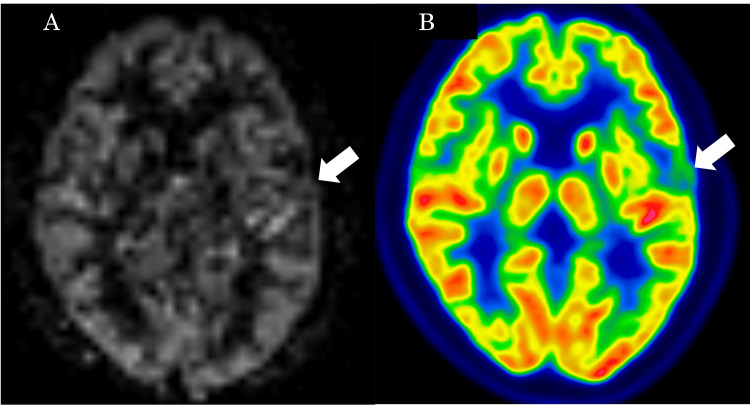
The ASL and FDG-PET scan Pseudo-continuous ASL (A) shows no obvious hyperperfusion within the tumor, and FDG-PET (B) shows no abnormal FDG uptake with a maximum standardized uptake value (SUV) max of 4.73 in the tumor (arrows). ASL: Arterial spin labeling, FDG-PET: Fluorodeoxyglucose positron emission tomography
